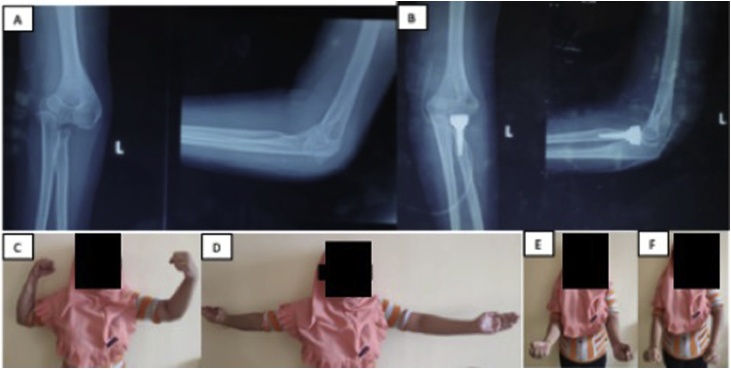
A Pre-operation AP/Lateral X-Ray of the left elbow from the third patient. The radial head was displaced >2 mm and conservative treatment would likely to fail, so the patient undergo surgery. Fig. 3B Post-operation AP/Lateral X-Ray of the left elbow from the same patient. The patient was also treated with radial head arthroplasty. Fig. 3C–F Active ROM evaluation of the same patient; elbow flexion of 80°; elbow extension of 10°; elbow supination of 80°; elbow pronation of 20°.
